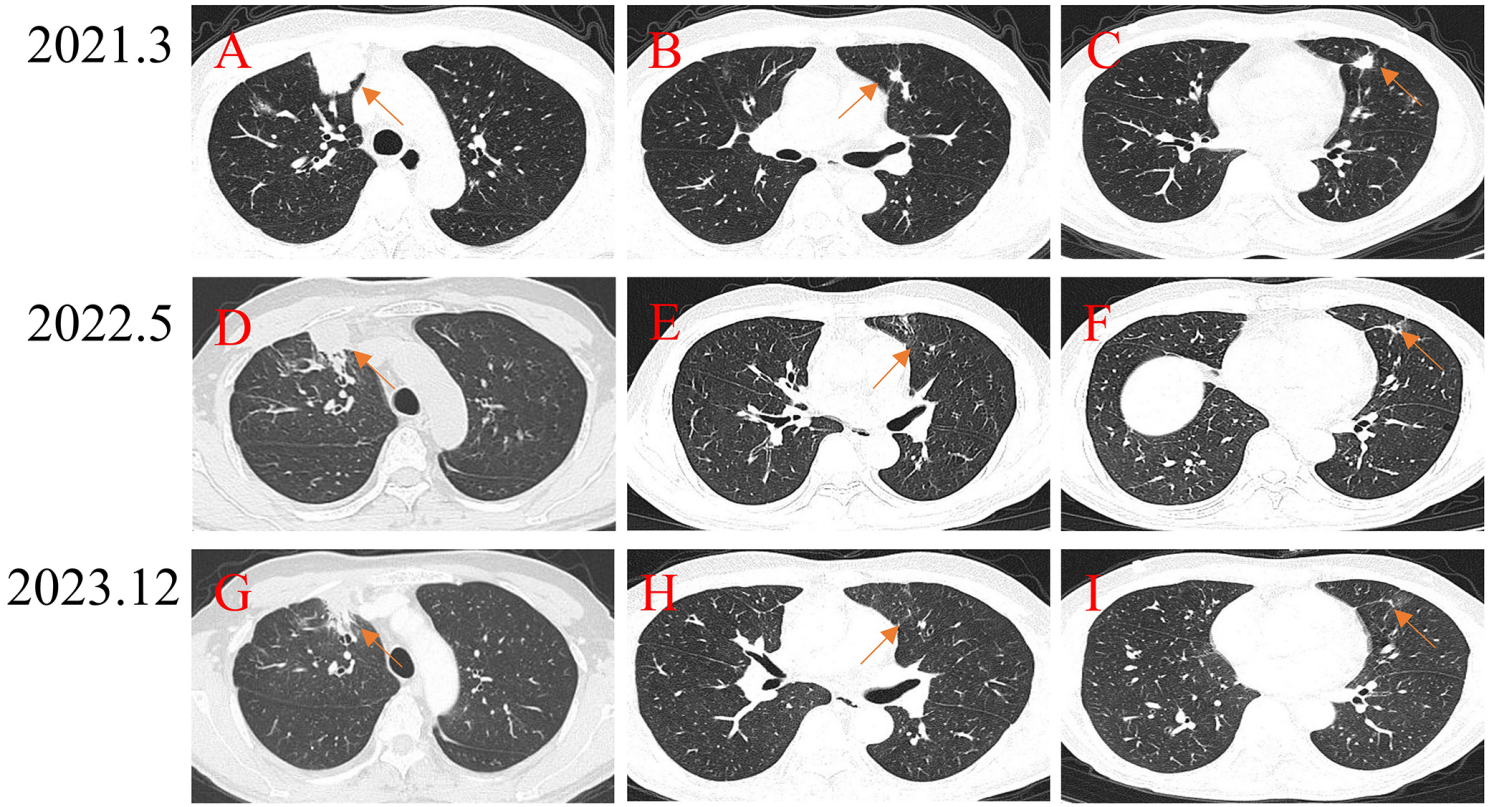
Treatment of a patient with high-grade UPS of the right thigh with lung metastases using PD-1 inhibitor + chemotherapy. (A-C) Pretreatment CT scan shows multiple lung metastatic lesions. (D-F) After receiving PD-1 inhibitor-based combination therapy, the tumor continuously shrinks. (G-I) Seventeen months after stopping PD-1 inhibitor-based combination therapy, the tumors continued to shrink.
